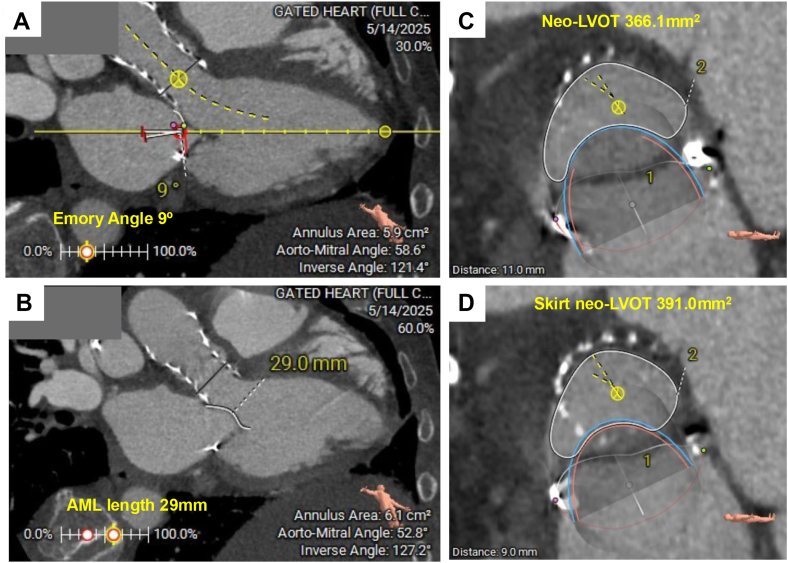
Preprocedural computed tomography. (A) Emory angle 9°. (B) AML length of 29 mm (C) Neo-LVOT of 366.1 mm^2^. (D) Skirt neo-LVOT of 391.0 mm^2^. AML, anterior mitral leaflet; LVOT, left ventricular outflow tract.
